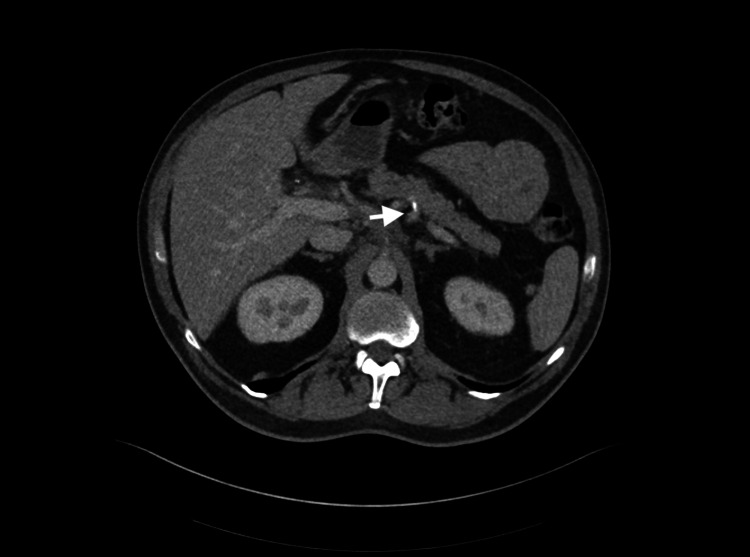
CT of the abdomen: mild haziness within the peripancreatic fat (white arrow) extending into the mesenteric root.
